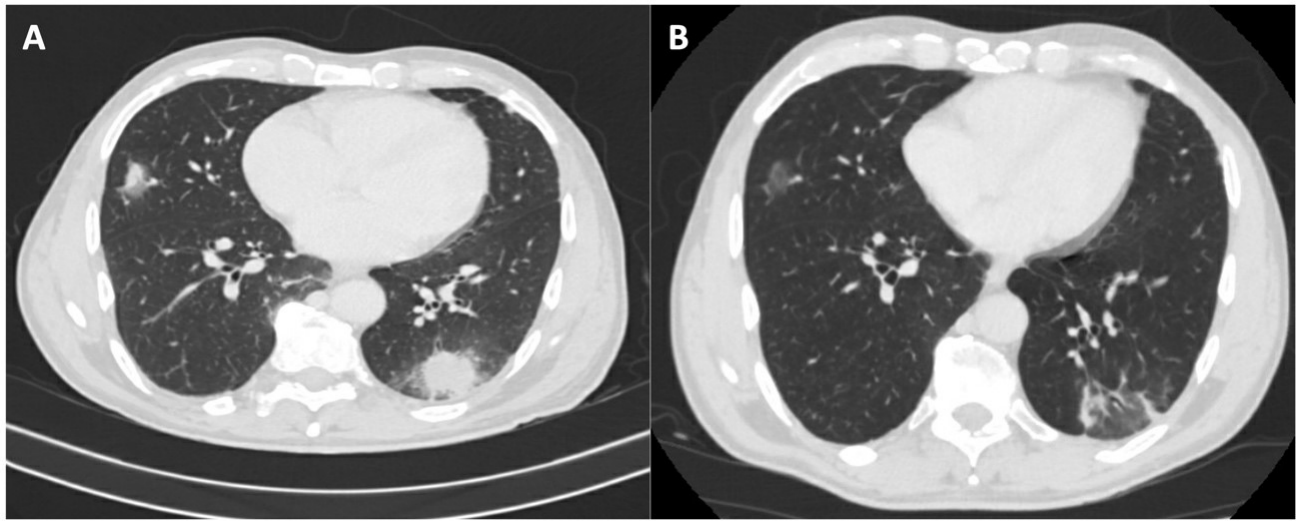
A: CT scan on patient admission with bilateral pulmonary infiltrates with halo sign; B: CT scan 3 months after discharge with reduction of pulmonary infiltrates
